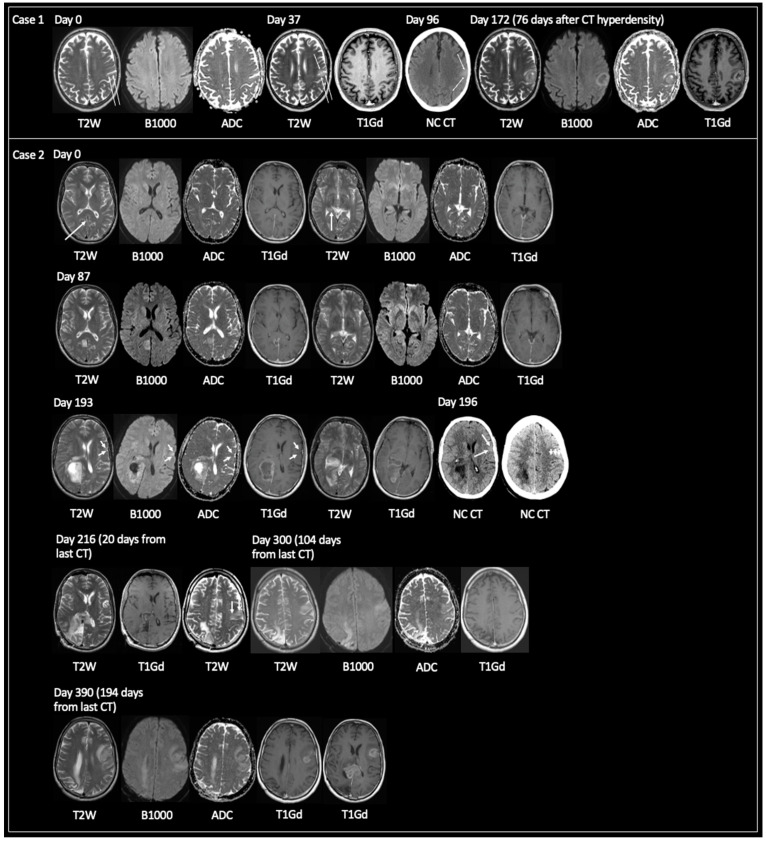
Examples of early GBM progressing through T2 hyperintensity to T2 iso and CT hyperdensity prior to showing TRA GBM. Case 1: 68-year-old male presented with dysphasia at day 96 leading to unenhanced CT investigation, which showed a solitary hyperdense lesion in the anterolateral aspect of the left parietal lobe, bordering the left supramarginal gyrus. MRI at day 172 showed TRA GBM with central necrosis and peripheral enhancement. MRI at day 0 obtained for an 8-week history of left-sided hearing loss revealed subtle cortical expansion at the site of future GBM (arrows) with no reduced diffusivity. On MRI day 37 the cortical expansion was more apparent showing relative growth to day 0. Case 2: 59-year-old male with progressively worsening headaches. MRI day 0 showed small foci of T2 signal hyperintensity in the cortex of the right precuneus (small arrow) and within the posterior aspect of the right parahippocampal gyrus (long vertical arrow). Neither the focus of T2 signal hyperintensity showed reduced diffusivity nor enhancement. Note the smooth dural thickening and enhancement on the T1Gd images. MRI day 87 showed interval growth of the lesion in the right precuneus with some central necrosis and peripheral enhancement. The lesion in the right parahippocampal gyrus had also grown relative to day 0 and continued to show facilitated diffusion and nonenhancement. The working diagnosis at the time of day 0 and day 87 was granulomatosis with polyangiitis, as the patient was ANCA positive and had no other known cause for dural enhancement. MRI day 193 showed growth at both lesion sites with TRA GBM. Note the new small ovoid focus of cortical T2 iso in the left inferior frontal gyrus (arrows), showing concomitant reduced diffusivity but no enhancement. An unenhanced CT obtained 3 days later, on day 196, showed corresponding CT hyperdensity in the lesion in the left inferior frontal gyrus and this subsequently developed into TRA GBM on MRI day 216. Note also the cortical T2 iso-lesion laterally in the posterior aspect of the left frontal lobe on MRI day 216 (3 vertical arrows), not clearly represented on CT day 196 (3 vertical arrows). This subsequently progressed to TRA GBM on MRI day 390 after showing growth and T2 iso signal on MRI day 300 with concomitant high B1000 signal but without enhancement (MRI day 300 was obtained 2 week after the patient’s third cycle of adjuvant TMZ—no RT was given as the patient declined whole brain RT, which was deemed the only valid RT option). Additionally, note TRA GBM within the splenium of the corpus callosum on MRI day 390 representing local progression of the original lesion (3 weeks following the patients sixth cycle of adjuvant TMZ). TRA GBM—typical radiological appearance of glioblastoma, NC CT—non-contrast CT, C+ CT—intravenous contrast-enhanced CT, T2W—T2-weighted, B1000—DWI, ADC—apparent diffusion coefficient map of DWI, T1Gd—T1-weighted post-gadolinium, RT—radiotherapy, TMZ—temozolomide, ANCA—antineutrophilic cytoplasmic antibody.
